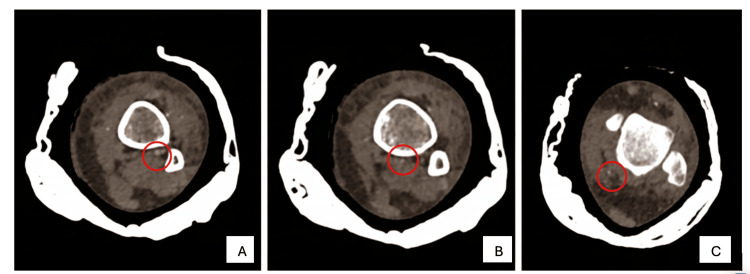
CT scan axial view Axial plane CT angiography from proximal (A) to distal (C) of the right leg showing absence of the PTA and a dominant peroneal artery (circled). PTA, posterior tibial artery
